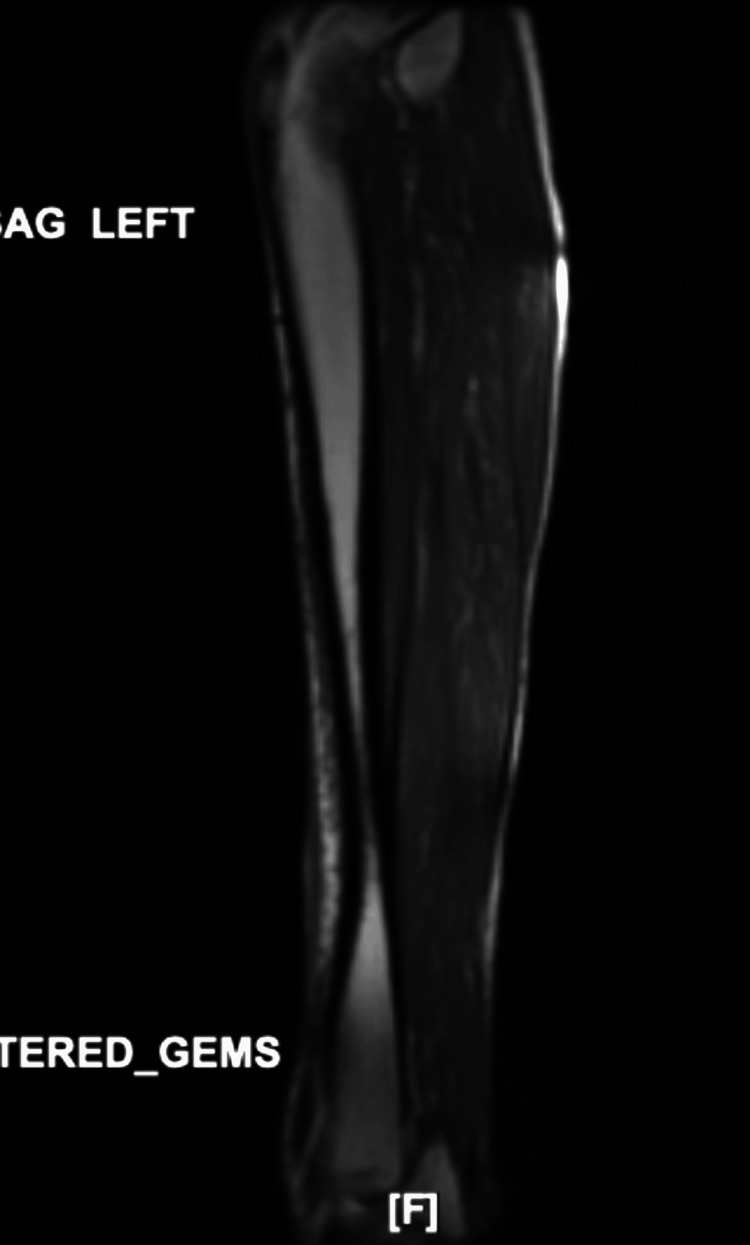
MRI of left tibia/fibula without contrast MRI: magnetic resonance imaging
